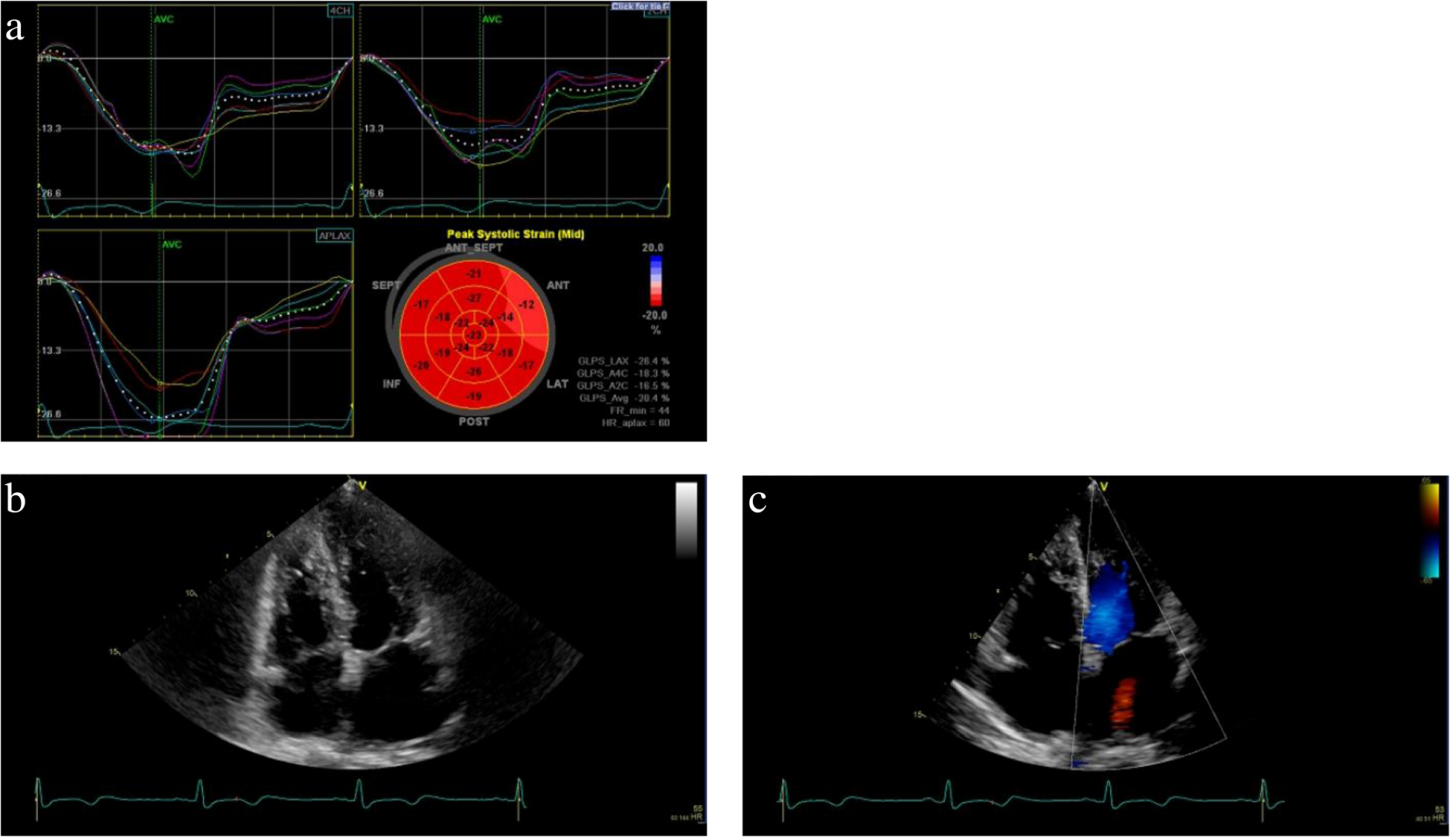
Transthoracic echocardiographic images obtained at 1 month after admission indicate (a) the recovery of left ventricular wall motion, (b) improvement of tethering, and (c) disappearance of mitral regurgitation
